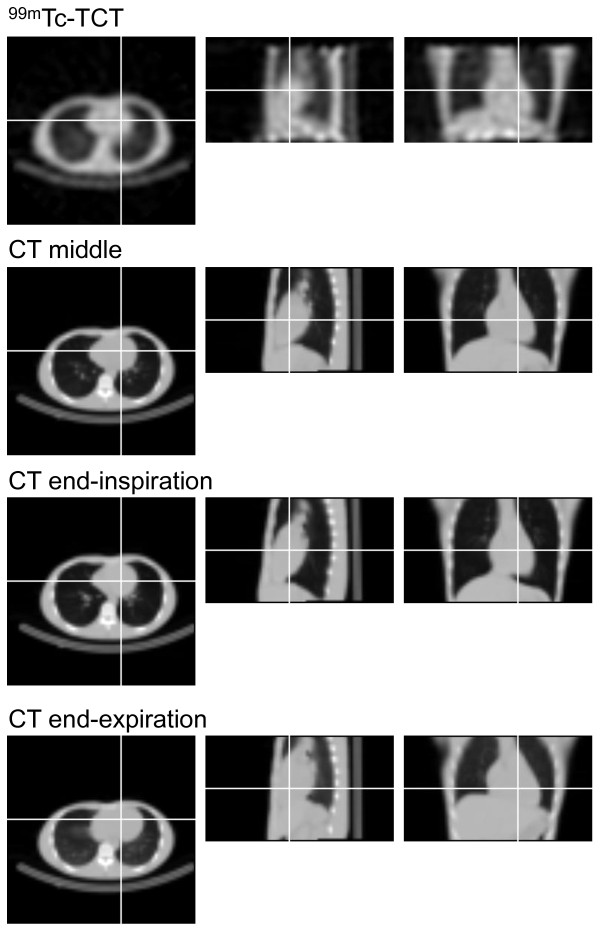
Attenuation map images of^99m^Tc-TCT and CT at three different respiratory phases. The left, middle, and right columns represent transaxial, sagittal, and coronal views, respectively.
